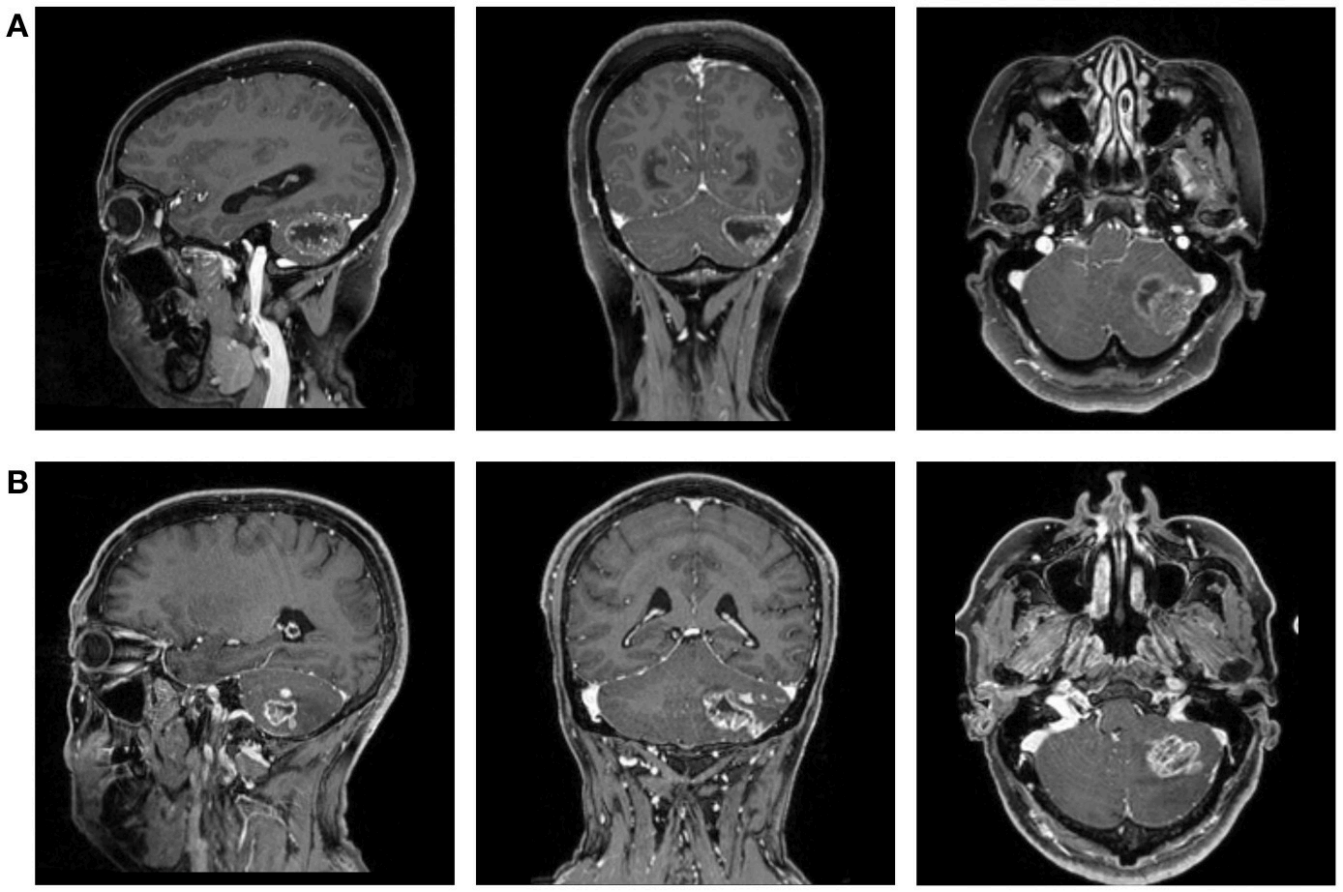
The magnetic resonance images (T1C) of a patient with (A) GBM and (B) MBTs.
